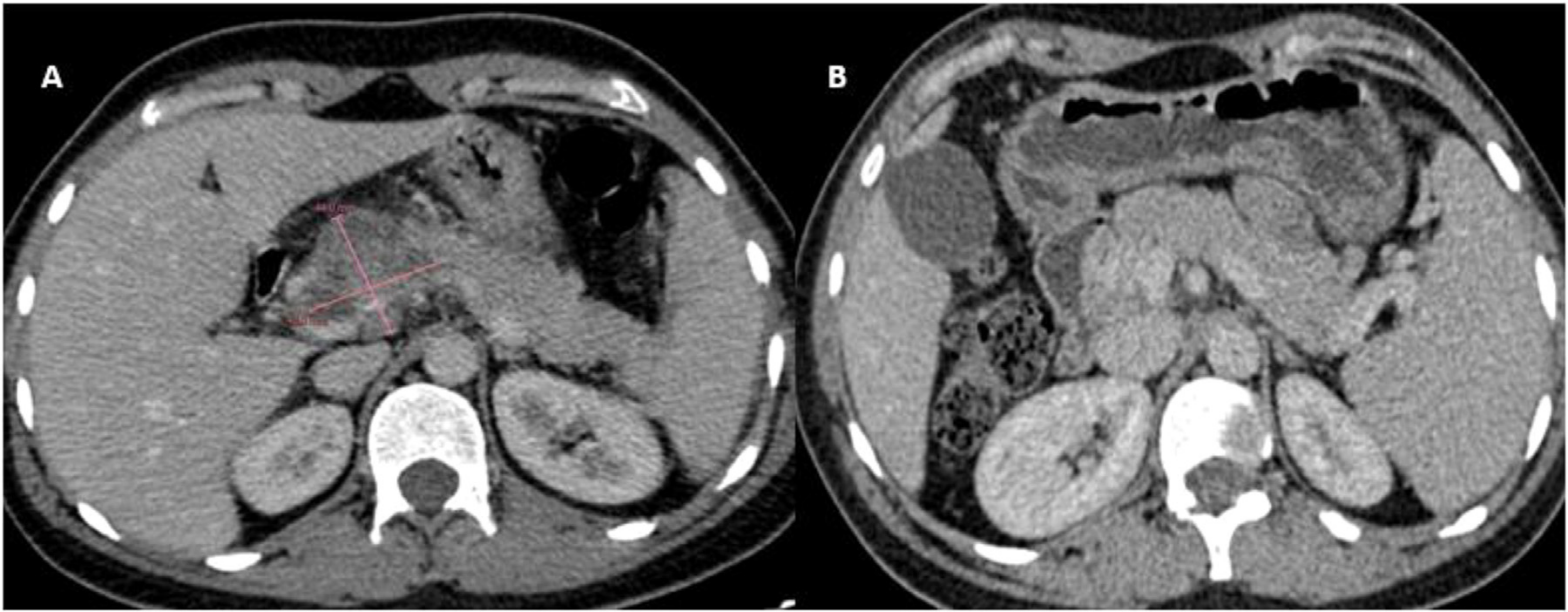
CT scan of the second case, transverse section showing (A) the pancreatic mass before anti-tuberculosis treatment, and (B) the pancreas after treatment.
